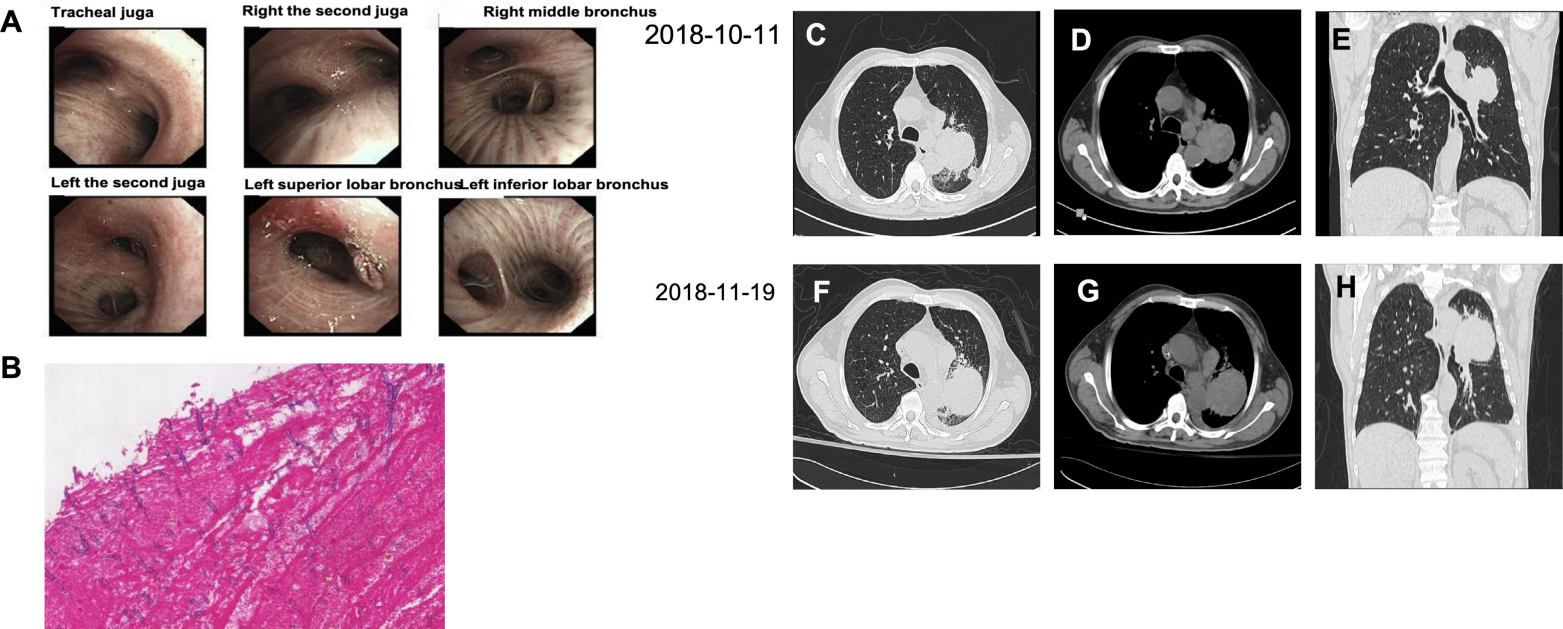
(A, B) Bronchoscopy and endobronchial biopsy. (C–H) Chest CT images showing tumour progression in the left lung following voriconazole therapy.
